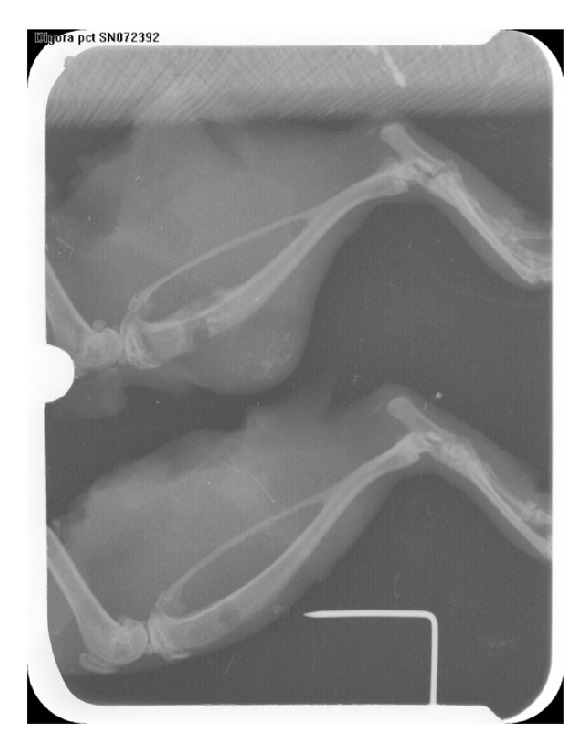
Radiographic view on day 14. A: Right tibia. B: Left tibia.
